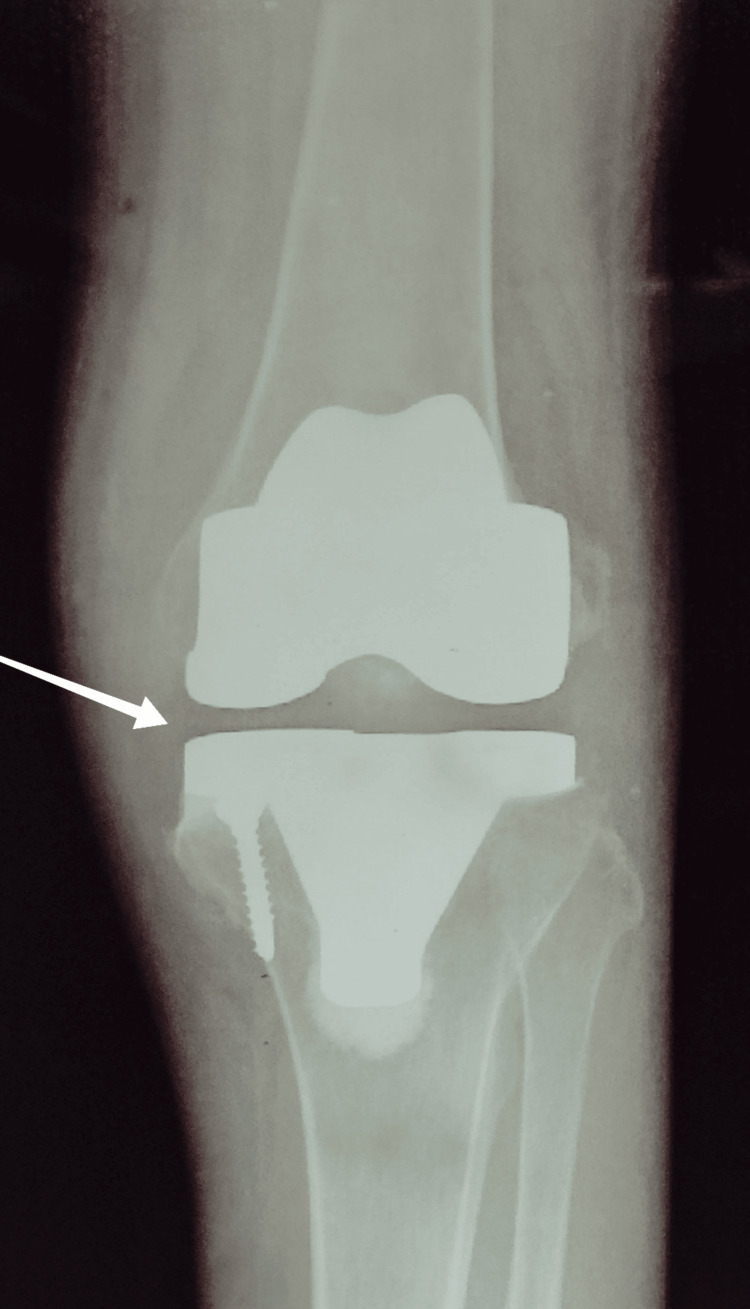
Anteroposterior X-ray view of the left knee following surgery.
